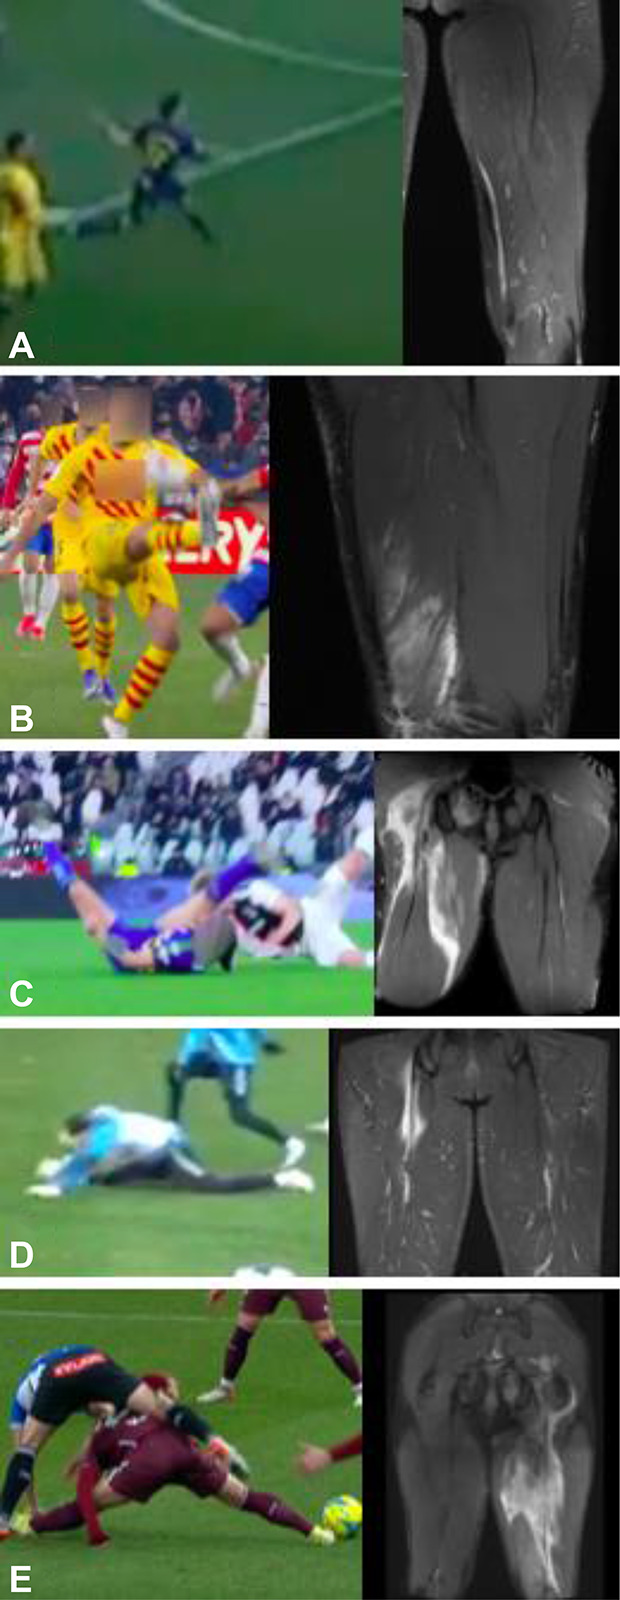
Assumed injury frames and MRI findings in stretch-type injuries. A, Case 8, distal SM injury. B, Case 11, distal BF injury of the MTJ. C, Case 12, proximal BF + ST avulsion. D, Case 13, proximal SM injury. E, Case 14, proximal BF + ST avulsion. MTJ, myotendinous junction
